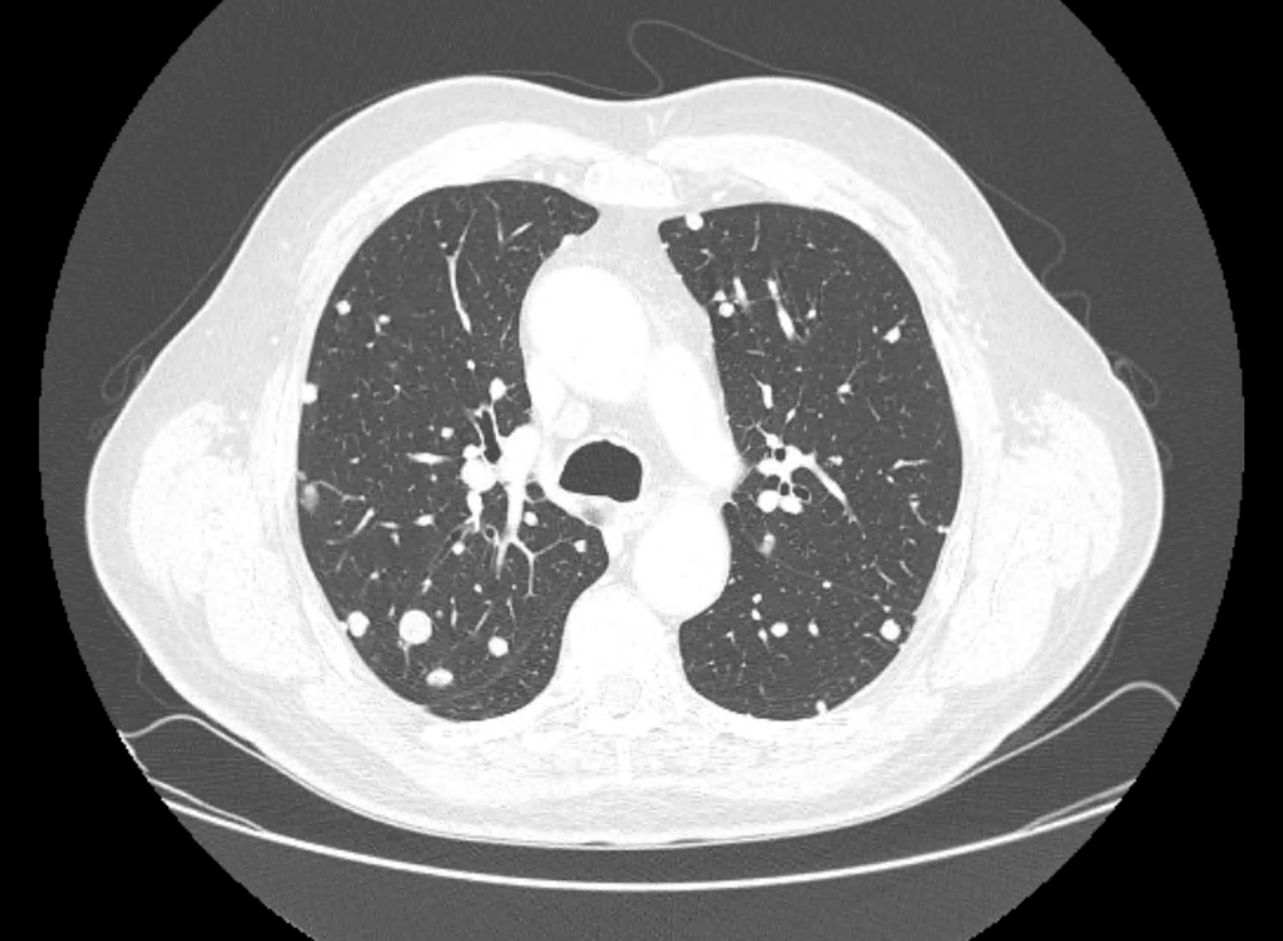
Follow-up chest CT after 10 months on apalutamide (Case 1) Extensive pulmonary metastases displaying a “balloon-release” pattern.
